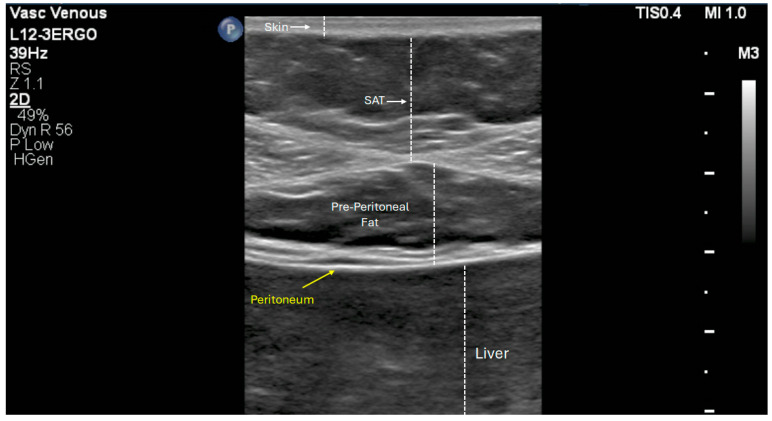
A transabdominal ultrasound image demonstrating pre-peritoneal fat in addition to subcutaneous and visceral adipose tissue: A transabdominal gray-scale ultrasound image of the midline upper abdomen acquired with a high-frequency (low-depth) ultrasound probe in a transverse position. Subcutaneous adipose tissue (SAT) is measured from the deep margin of the skin to the linea alba (yellow arrow). The linea alba can be identified as the bright echogenic tissue connecting the darker striated muscle bellies of the rectus abdominis muscles. Pre-peritoneal fat is the fat pad between the linea alba and the peritoneum (the deeper echogenic linear structure superficial to the liver). Pre-peritoneal fat thickness has also been identified as a potential imaging marker correlating to metabolic-related cardiovascular diseases. Figure courtesy of Jordan Perchik MD.
